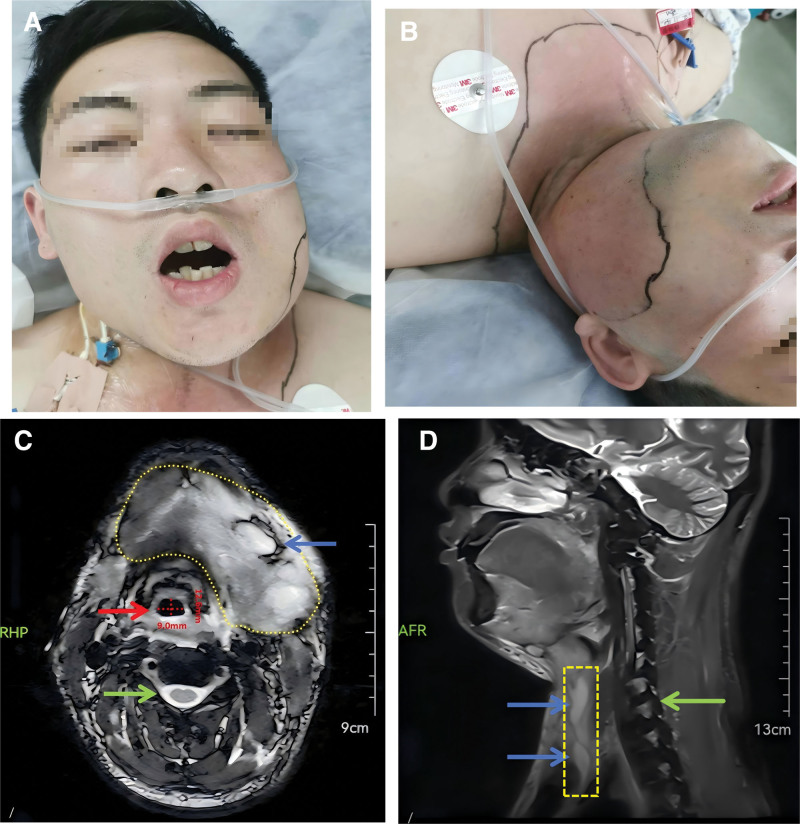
(A, B) Pre-anesthesia induction assessments reveal significant trismus and erythematous, edematous maxillofacial regions. (C, D) Magnetic resonance imaging (MRI) demonstrates abscess cavities (blue arrows), the surrounding swollen tissues (yellow areas), and the cervical vertebrae (green arrows) in both the axial (C) and sagittal (D) sections. The axial view (C) of the MRI reveals a compressed trachea (red arrow) with an anteroposterior diameter of 9.0 mm and a transverse diameter of 12.6 mm. MRI diagnosis: (1) Multiple abnormal signal shadows were observed in the left submandibular gland region, submandibular space, and adjacent to the thyroid gland. Subcutaneous exudation was noted in the left neck and submental area, suggestive of an infectious process. (2) There is evident swelling of the left lateral pterygoid muscle and masseter muscle.
